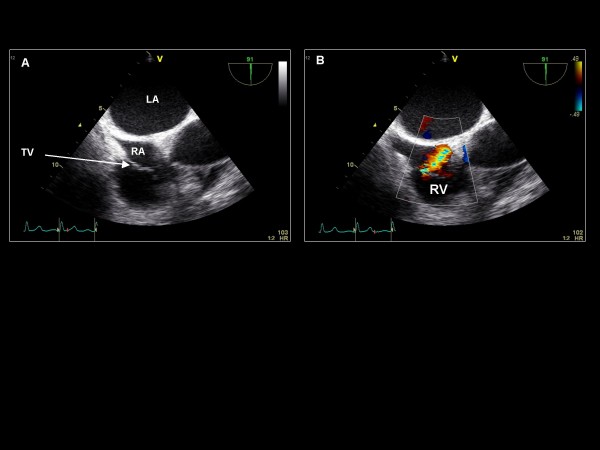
Imaging plane 90°, (A) The TV, RA, and interatrial septum are visualized. (B) Color Doppler is applied to the tricuspid valve demonstrating mild tricuspid regurgitation.
